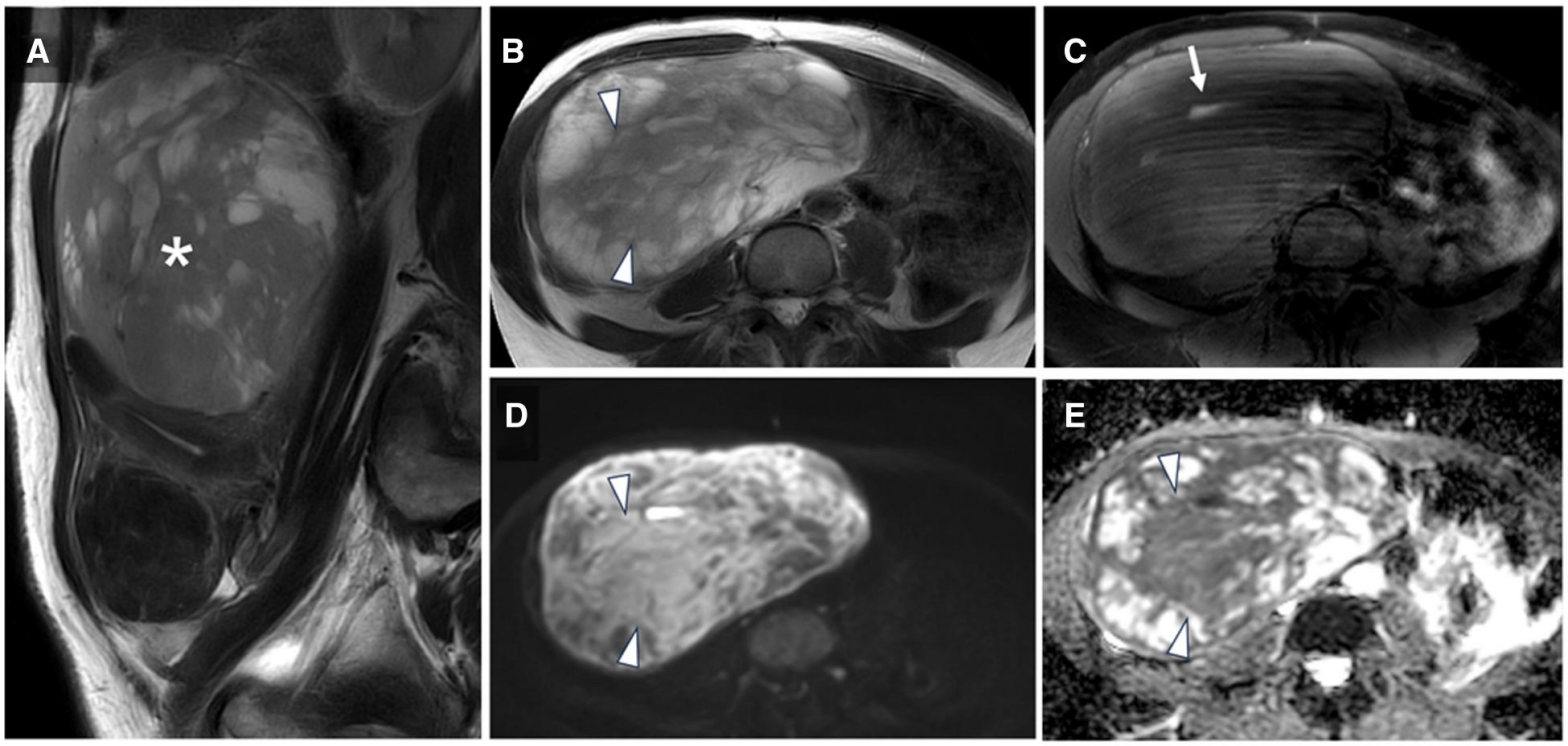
Initial MRI findings from the previous hospital. (A) Sagittal T2-weighted image (T2WI) showing a subserosal mass (*) with a maximum diameter of 15 cm located in the posterior wall of the uterus. The mass demonstrates mild hyperintensity and contains multiple cystic changes. (B) Axial T2WI showing a mildly hyperintense mass with cystic changes in the right lower abdomen. (C) Axial fat-suppressed T1-weighted image showing areas of hyperintensity within the mass, suggesting haemorrhage (white arrow). (D) Axial diffusion-weighted image (DWI) showing the mass with high signal intensity, consistent with its mild hyperintensity on T2WI, while the cystic areas appear hypointense. (E) Axial apparent diffusion coefficient (ADC) map showing the mass with low signal intensity, indicating restricted diffusion, while the cystic areas appear hyperintense, consistent with no restricted diffusion (white arrowhead).
